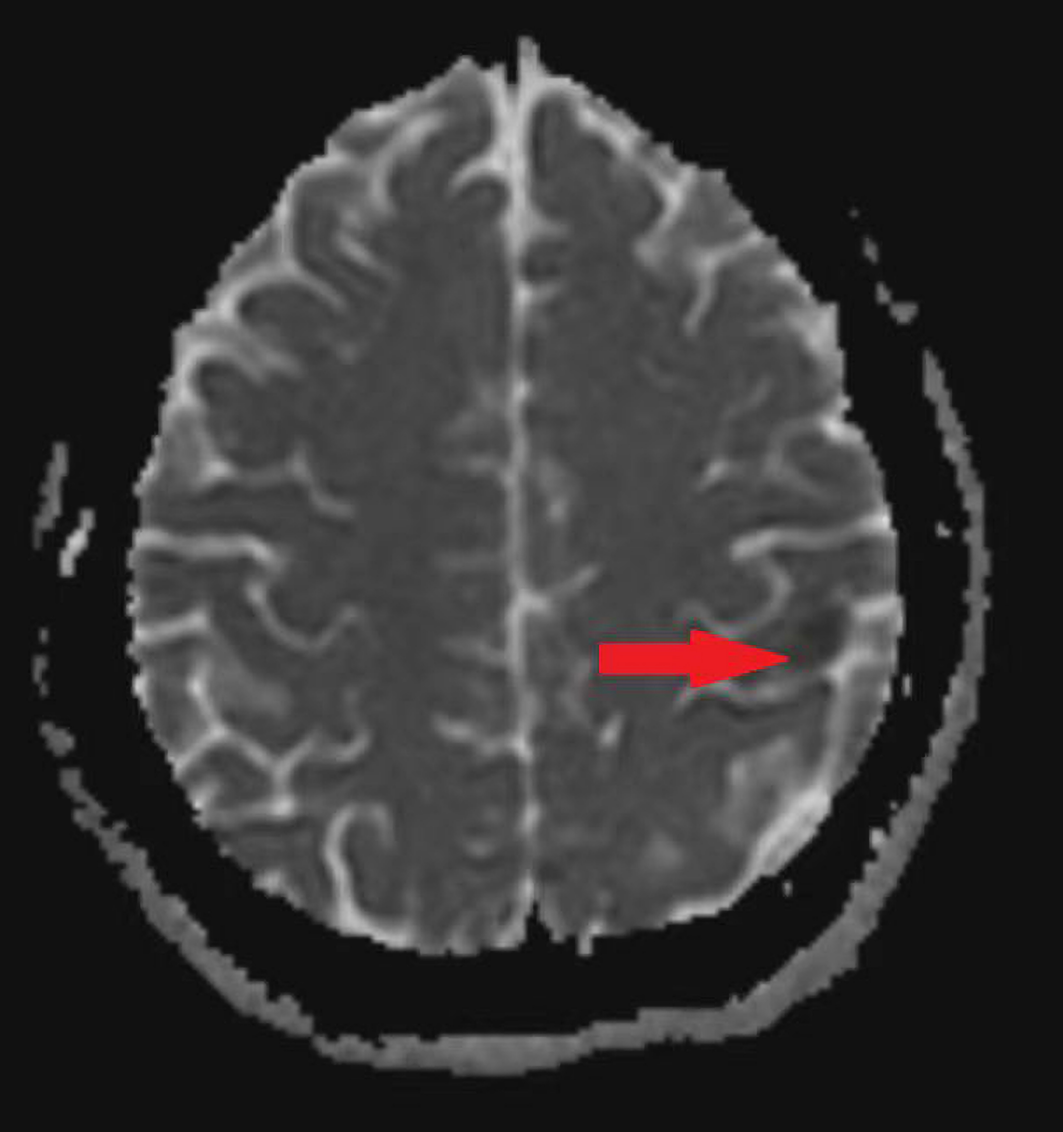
The MRI of the patient's head before operation, the arrow is the focus area.
